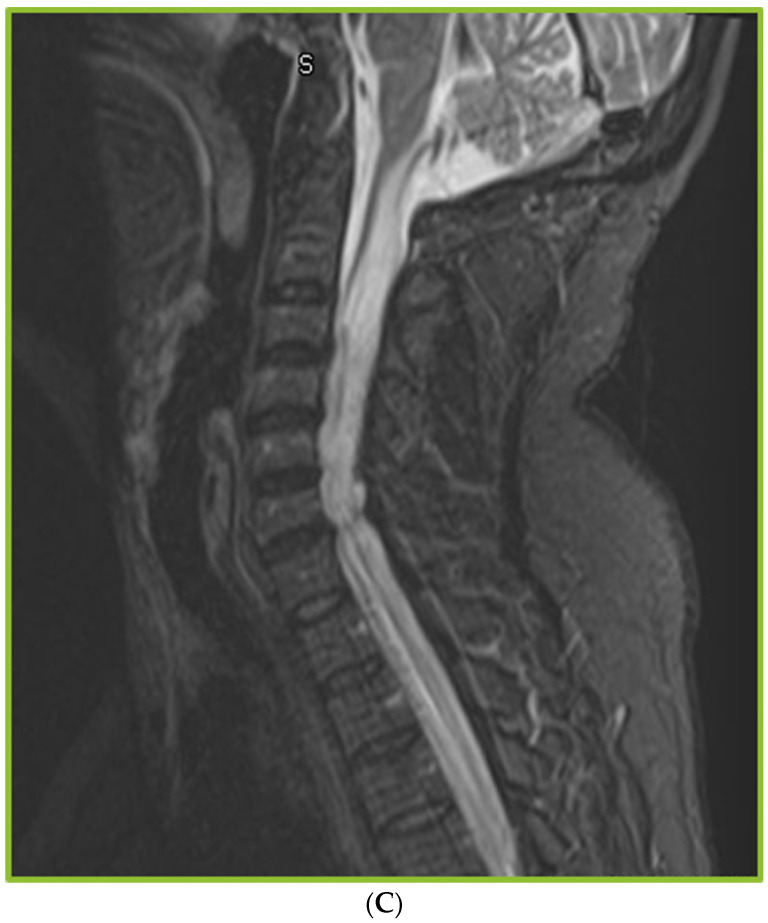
(A) Sagittal thoracic MRI spine: Septations, ill-defined irregularity in the intrathecal space, deformity of the thoracic cord, complex ill-defined CSF collections. (B) Sagittal post contrast MRI cervical spine: Extensive multilocular fluid tracking from the cervical medullary junction to C5 level with marginal rim enhancement. (C) Sagittal cervical and thoracic MRI spine: Extensive syringohydromyelia present throughout the entire cervical cord and upper thoracic cord.
